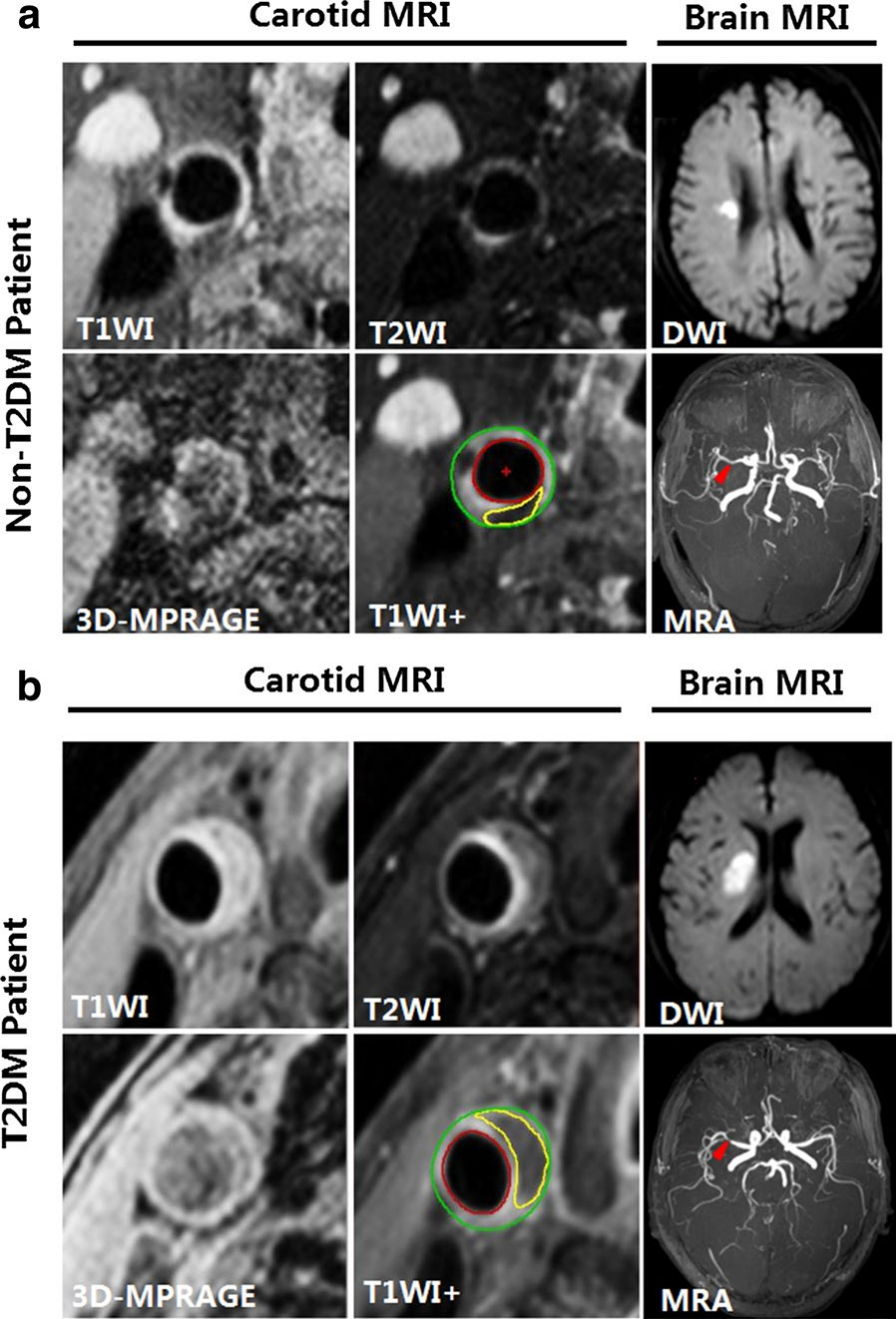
Representative MR images of a T2DM and a non-T2DM subject. a An atherosclerotic plaque with small LRNC (*yellow outline*) is detected in the right carotid artery of a non-T2DM patient: iso-intensity on T1WI and T2WI; no enhancement in T1WI+; hypo-intensity on MP-RAGE image. Cerebral DWI demonstrates a small perforating artery infarct (hyper-intensity) at right hemisphere. Brain MRA demonstrates right MCA M1 segment with mild stenosis (*red arrow*). b An atherosclerotic plaque with large LRNC (*yellow outline*) with mild to moderate stenosis is detected in the right carotid artery of a T2DM patient. LRNC appears as iso-intensity on T1WI; hypo-intensity on corresponding T2WI; no enhancement in T1WI+; hypo-intensity on MP-RAGE image. Cerebral DWI demonstrates large volume of ACI (hyperintensity) at right hemisphere. Brain MRA demonstrates right MCA M1 segment with moderate stenosis (*red arrow*). *ACI* acute cerebral infarct, *LRNC* lipid-rich necrotic core, *WI* weighted image, *T1WI+* contrast enhanced T1-weighted image, *MCA* middle cerebral artery
